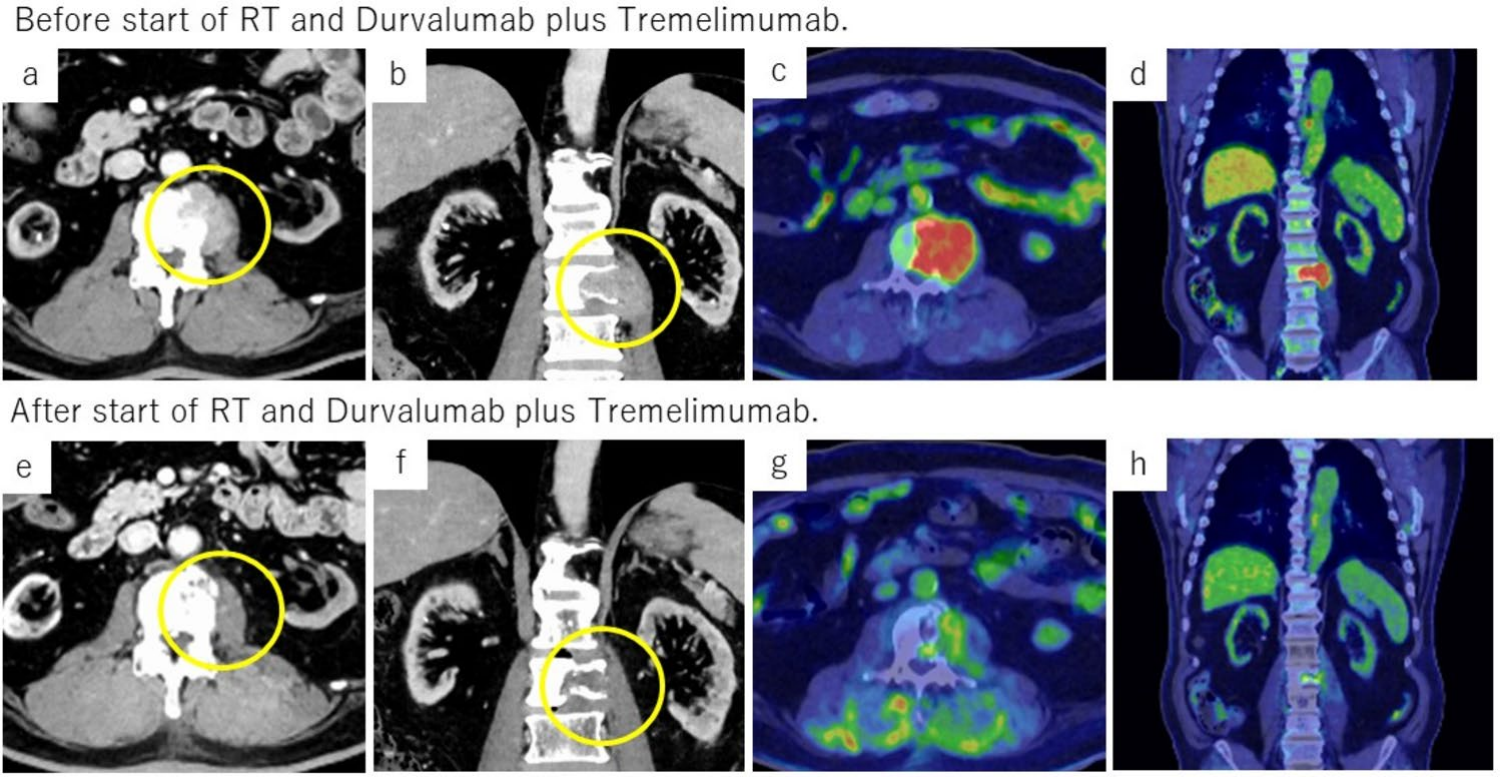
Contrast-enhanced computed tomography (CT) imaging test and Fluorine-18-fluorodeoxyglucose positron emission tomography (FGD-PET) imaging test results before and after treatment. a–d Before RT and durvalumab plus tremelimumab. e–h 9 months after RT and durvalumab plus tremelimumab, 8 courses of durvalumab
